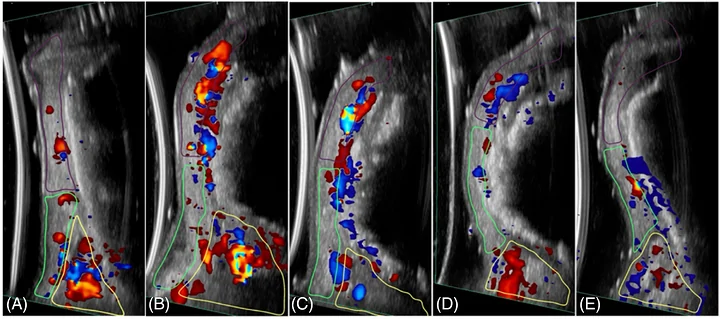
Ultrasound color flow velocity from baseline to 5‐month post‐op visits. Color pixels indicate per‐pixel mean blood flow velocity with respect to the transducer. Red and blue correspond to positive and negative velocities, i.e., to and from the transducer, respectively. (A) The baseline image shows sporadic blood flow in the keratinized mucosa (KM) (purple region of interest [ROI]) as well as lining mucosa (LM) (green ROI), with significant flow in the muscle layer (yellow ROI) compared to the KM, which might be the branches of mental vessels. (B) A similar pattern was observed on Day 3, KM displayed a significant increase in blood flow velocities, while the other two ROIs showed moderate increases. (C) On Day 10, significant blood flow was still present in KM. (D) and (E) Color flow velocity diminished progressively over time, to eventually return to baseline.
